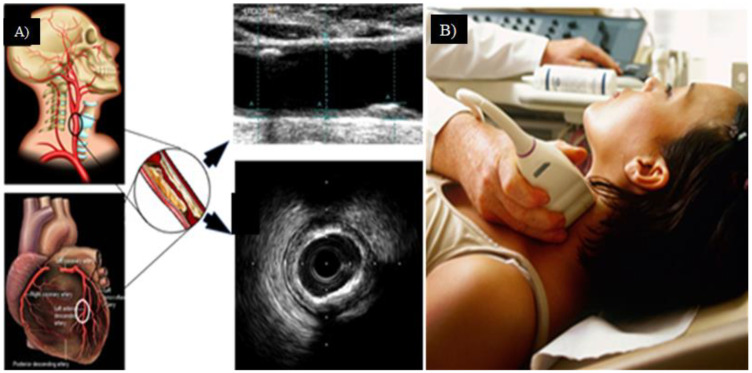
(A) CTAD is a potential surrogate marker for COAD, shown using an IVUS-based vascular cross-sectional scan. (B) B-mode carotid longitudinal imaging system using linear ultrasound [159].
